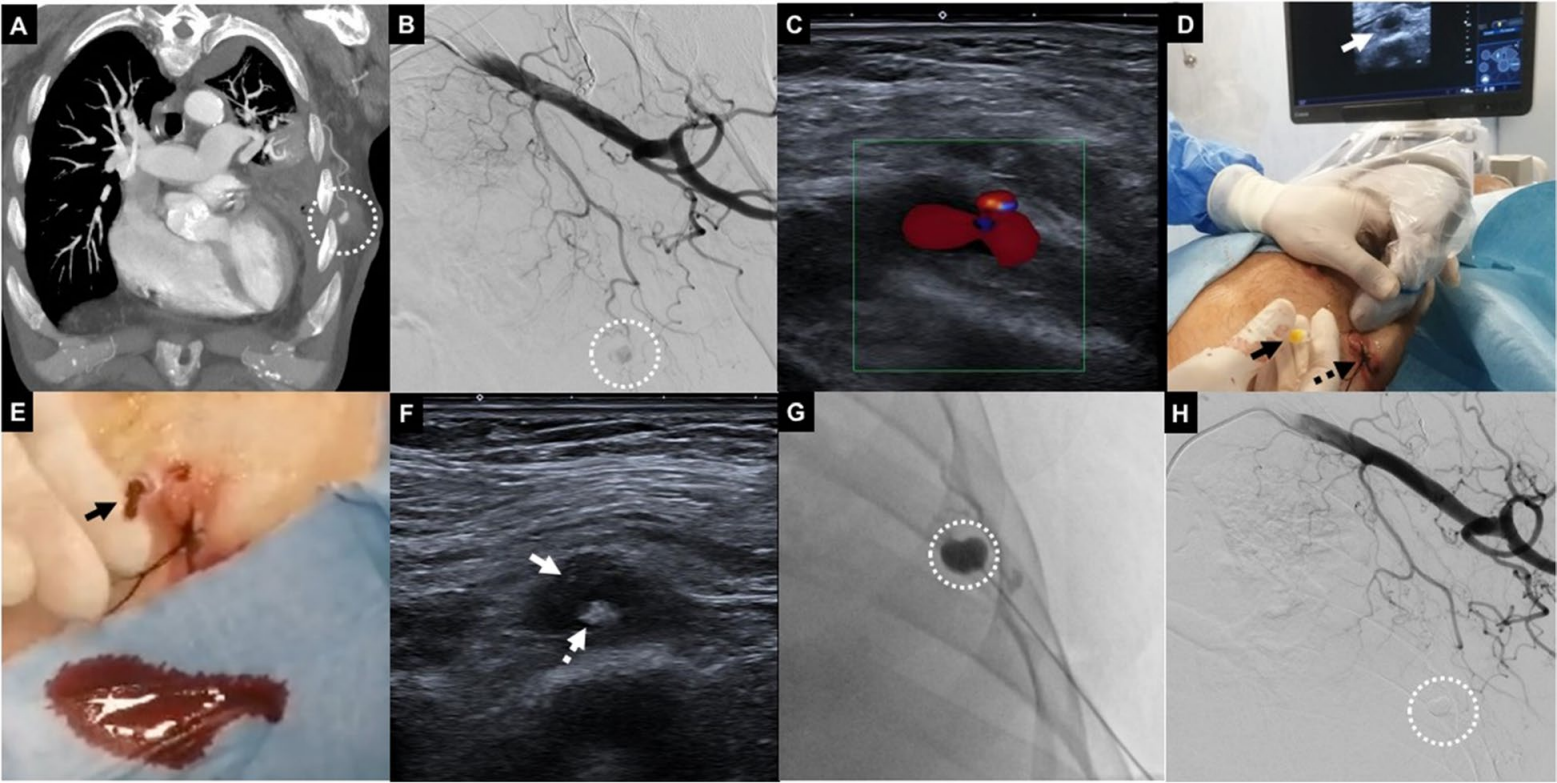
Seventy-one years old male with left thoracic wall iatrogenic bleeding after pleural drainage. A Coronal CT MIP reconstruction in arterial phase showing 14 mm PA (white dotted circle) refurnished by left thoraco-scapular branch. B Left succlavian DSA confirming the lesion (white dotted circle). C Color-US with linear probe identifying superficial PA. D Puncture of the lesion with a 20G 10 cm needle (black arrow) under US guidance (white arrow); skin suture of the previous drainage access is also evident (dotted black arrow). E After stylet removal, arterial blood sinked from needle hub (black arrow), confirming PA intraluminal needle tip positioning. F US showing proper intraluminal needle tip location (white arrow); initial glue cast formation is also evident (white dotted arrow). G Fluoroscopic monitoring of glue-Lipiodol injection into the PA (white dotted circle). H Final DSA confirming technical success, glue cast being appreciable (white dotted circle)
